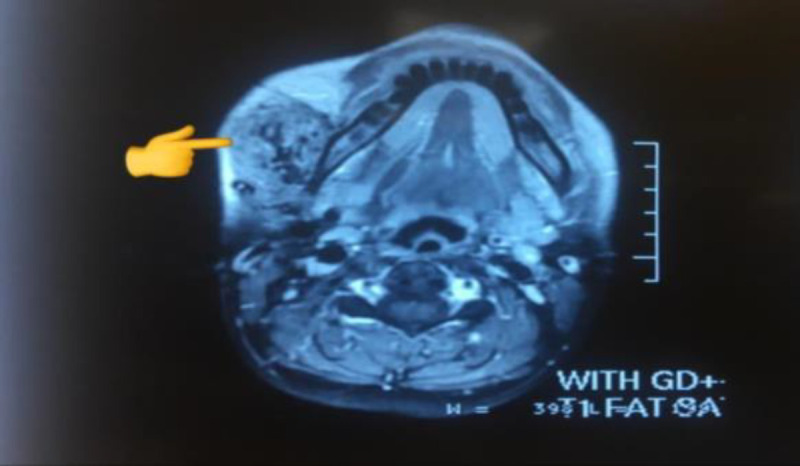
T1-weighted FAT-SAT axial sequence showing an irregular enhancement following the medium contrast uptake (Pointer)
